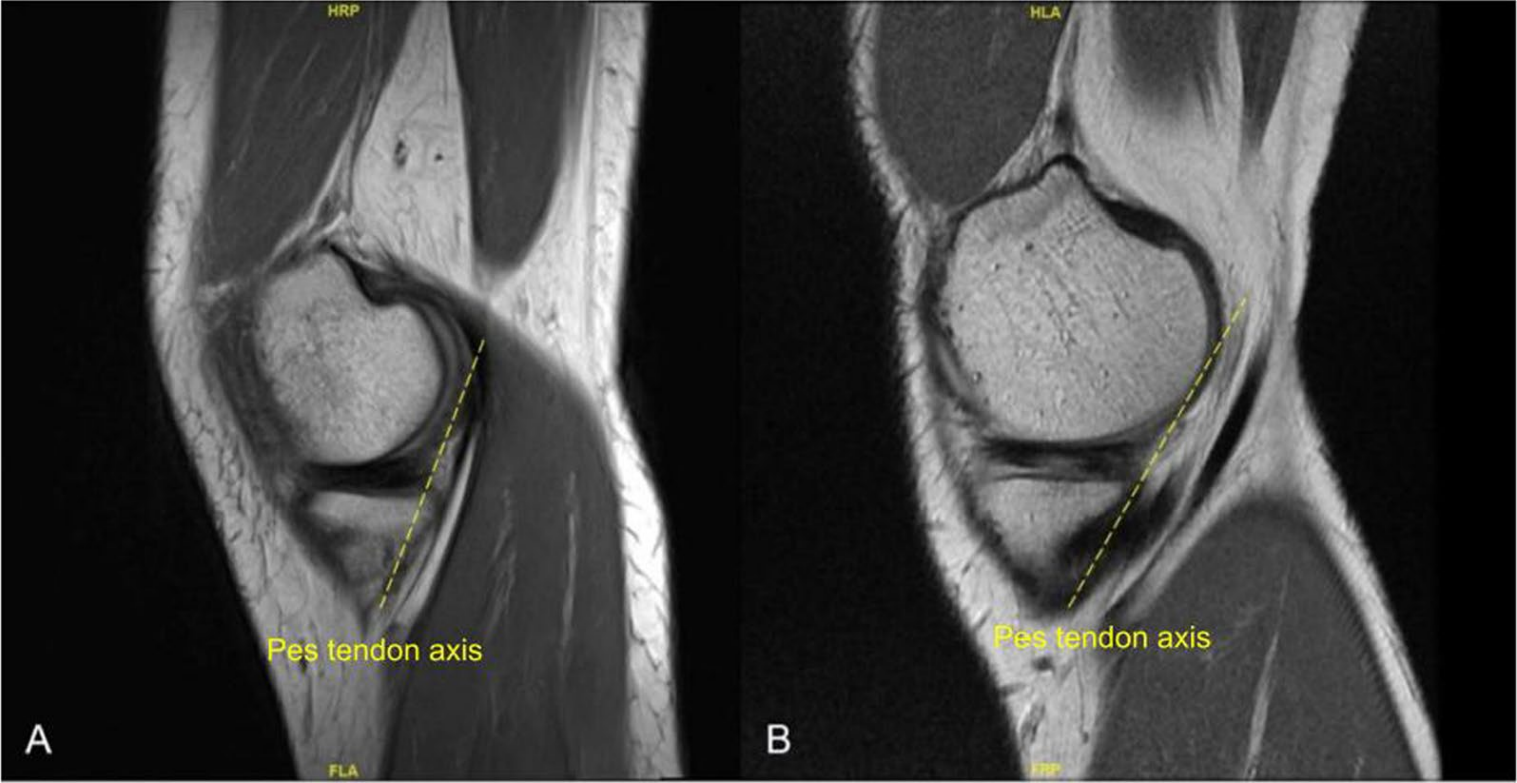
T2-weighted MRI showing sagittal cuts of the knee of two patients, demonstrating differing axes of pes tendons which are used as reference for subsequent measures
